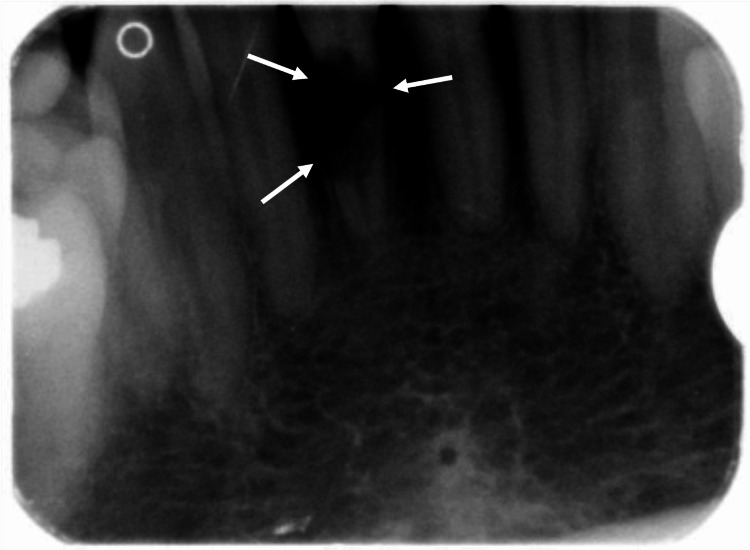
Periapical radiograph demonstrating extensive root resorption of the lower right central incisor prior to extraction.
